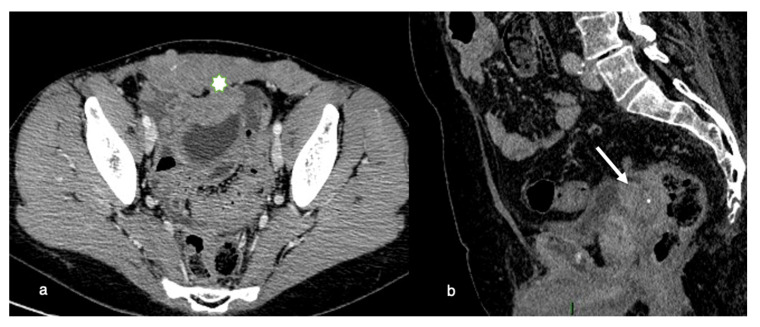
Pelvic involvement. (a) Axial contrast-enhanced CT image shows a diffuse and irregular thickening of the anterior pelvic wall (asterisk) caused by the extension of tumoral tissue to adjacent muscles with penetration into the abdominal wall (b) Sagittal contrast-enhanced CT image shows a nodular cancerous implant located in the recto-sigmoid pouch (arrow) invading the bladder posterior wall (trigone of the bladder).
